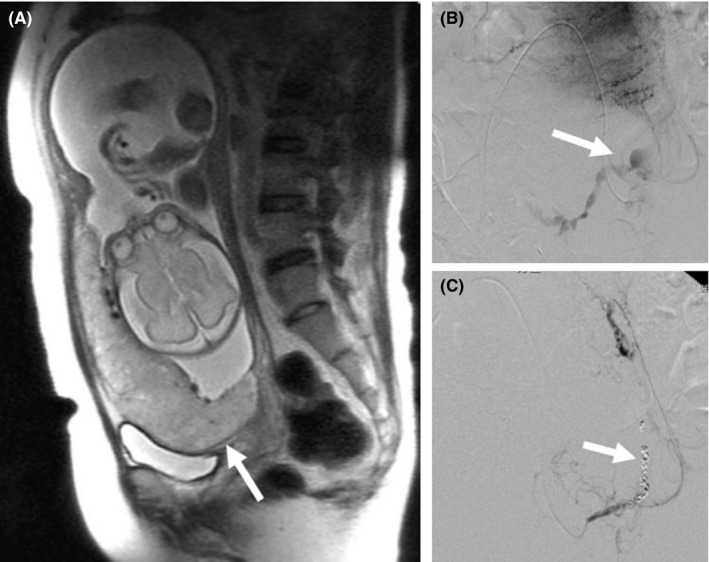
A, Sagittal T2‐weighted magnetic resonance image showing placenta previa at 28 wk of gestation. B, Pelvic angiogram showing extravasation from the left uterine artery (arrow) at postpartum hemorrhage after elective cesarean section for placenta previa at 36 wk of gestation. C, Platinum microcoil embolization of the left uterine artery (arrow) to achieve hemostasis after incomplete embolization by gelatin sponge particles
